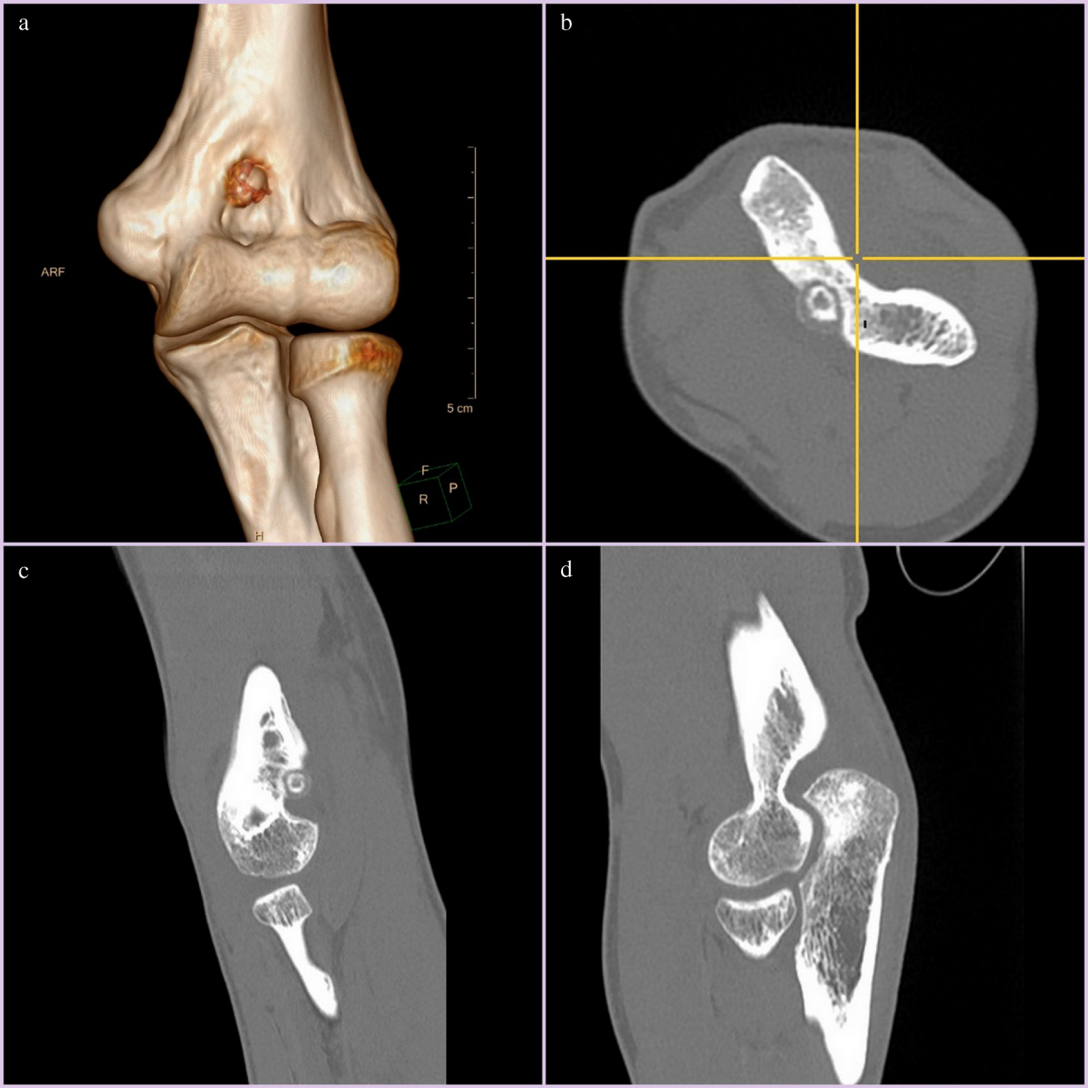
Computed tomography images showing the lesion in coronal 3D reconstruction (a), axial (b), and sagittal (c) views. (d) Shows an otherwise normal elbow joint.
